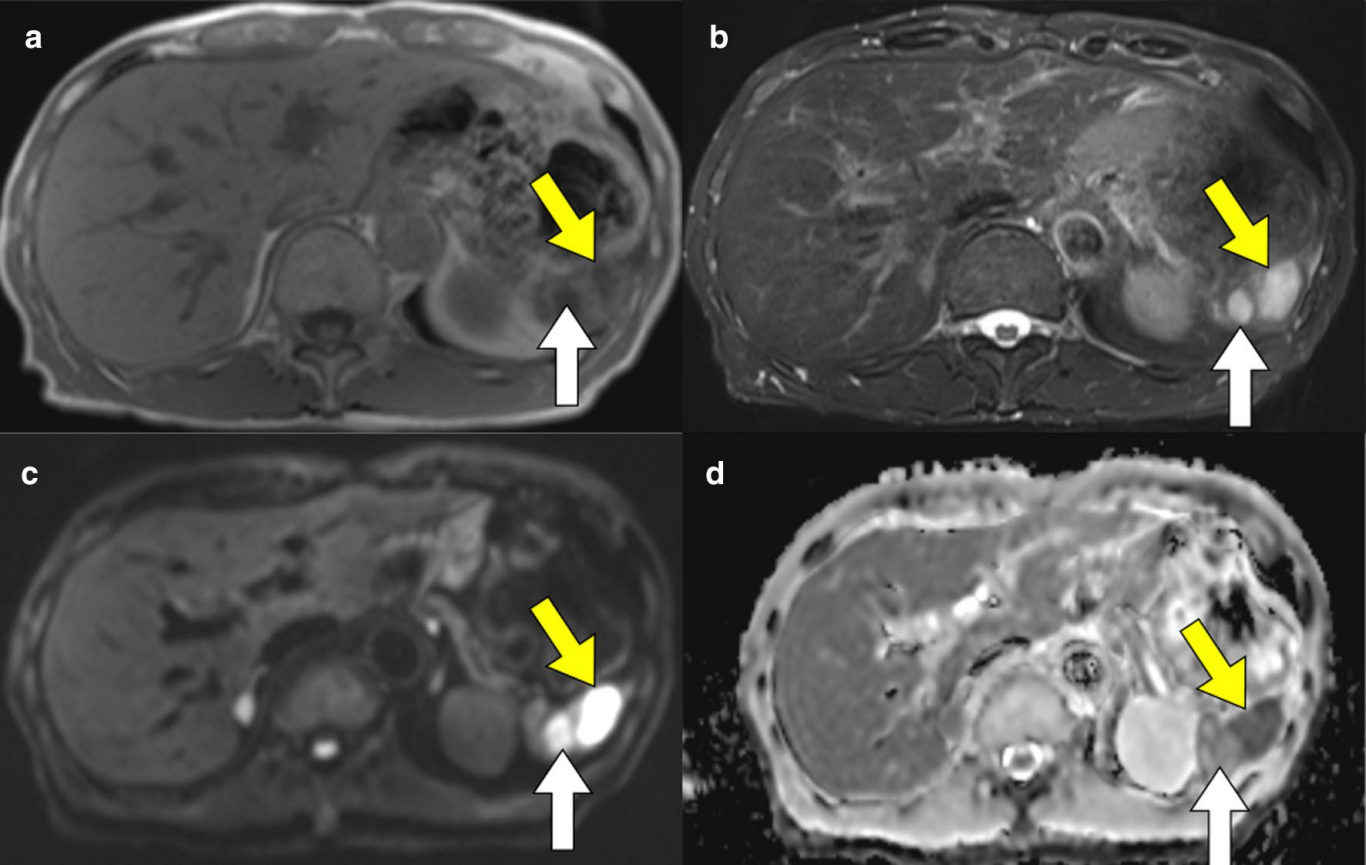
Magnetic resonance imaging findings. Magnetic resonance imaging revealed both the larger (yellow arrow) and smaller (white arrow) tumors as having low signal intensity on T1-weighted MRI (a), heterogeneous high intensity on T2-weighted MRI (b), and high signal intensity in diffusion-weighted imaging (c), and the apparent diffusion coefficient value revealed low signal intensity (d)
